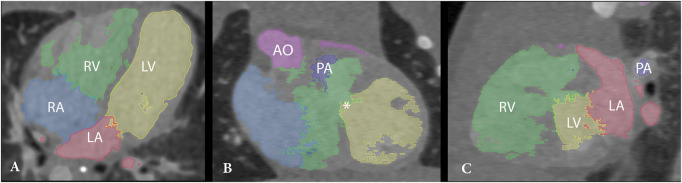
CT scan with segmentations of the cardiac structures. An axial (A), coronal (B) and sagittal (C) cross-sectional slice are shown, together with the highlighted cardiac segments. Ao: aorta; LA: left atrium; LV: left ventricle; PA: pulmonary artery; RA: right atrium; RV: right ventricle; ^: coronary artery; *: ventricular septum defect.
